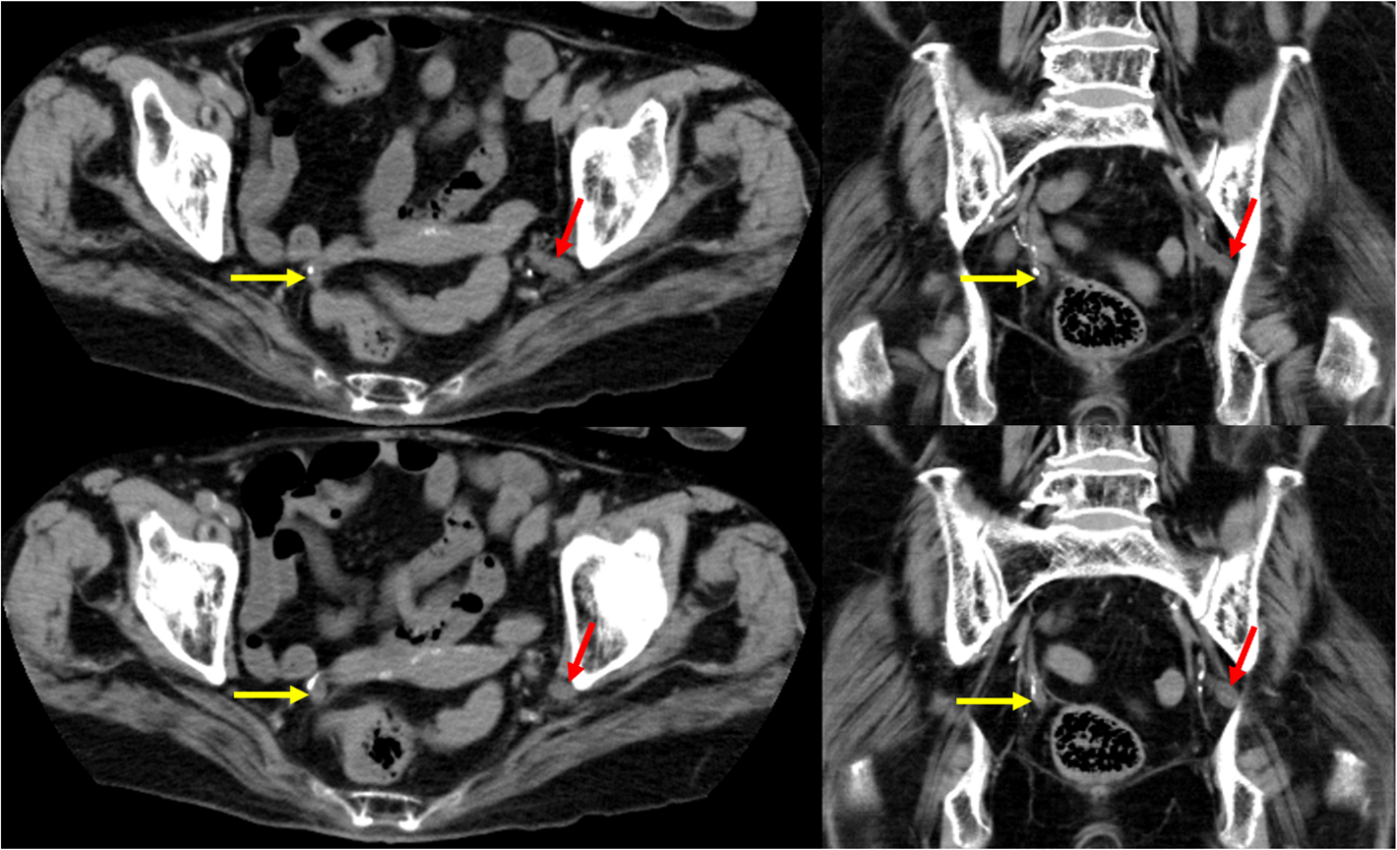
Computed tomography reveals deviation of the left ureter (red arrows) to the sciatic foramen. The right ureter is indicated by yellow arrows
